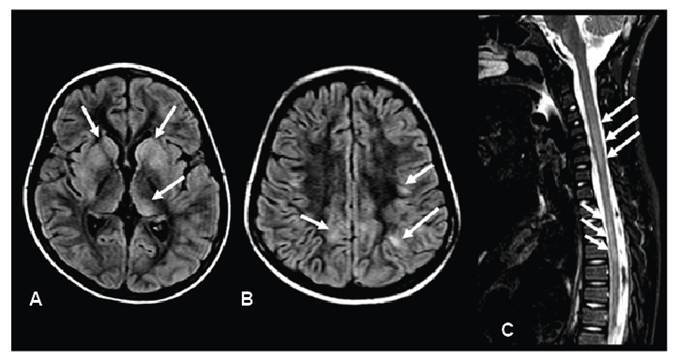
Brain magnetic resonance imaging (MRI) reveals multiple bilateral and asymmetrical lesions with hyperintense signal on FLAIR, involving the basal ganglia (arrows in A) and subcortical white matter (arrows in B), consistent with acute disseminated encephalomyelitis. Spine MRI demonstrates longitudinally extensive myelitis with hyperintense signal on T2-weighted imaging (arrows in C).
